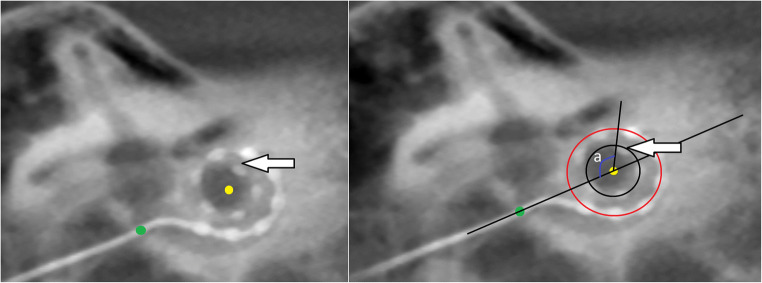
Cochlear view reformat CBCT image shows the measurement of the angle insertion of the electrode inside the cochlea. It equals the numbers of turns the deepest electrode contact (white arrow) forms inside the cochlea around a reference line (the line joining the center of the round window/cochleostomy (green dot) and the center of a circle formed by the 3 most apical electrodes (yellow dot)). The insertion angle measurement = 2 turns - angle (a) = 360° (red circle) + 360° (black circle) - angle (a)*Mid-modiolar view*: oblique view in a plane perpendicular to the cochlear view (Fig. 1) for assessing the scalar position of the CI electrodes (Fig. 4).
